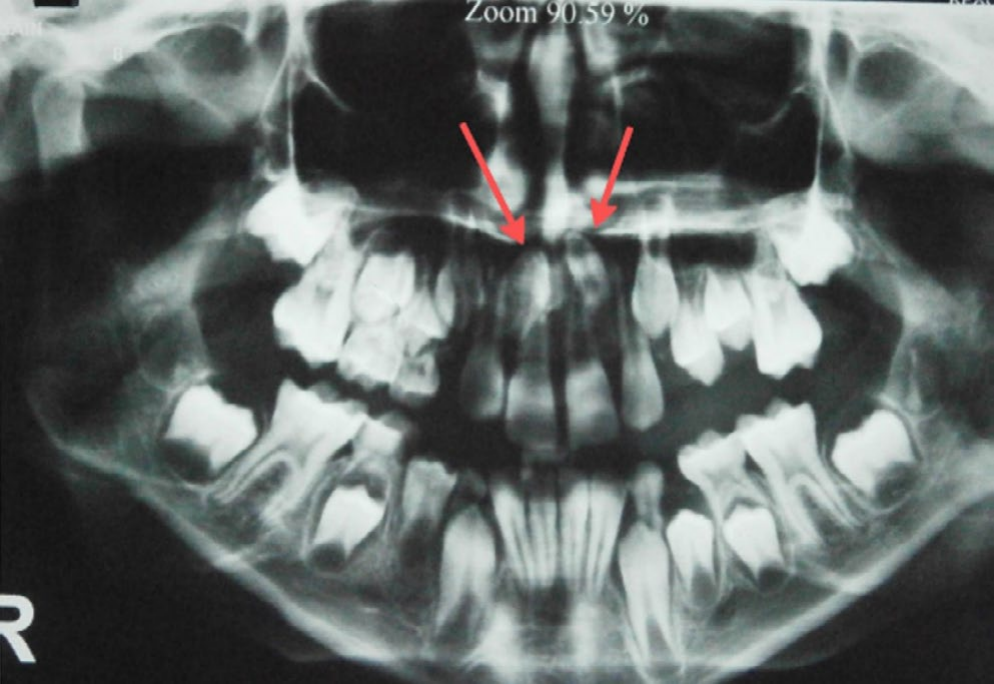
Panoramic radiograph showing deeply impacted supernumerary teeth in the anterior maxilla
